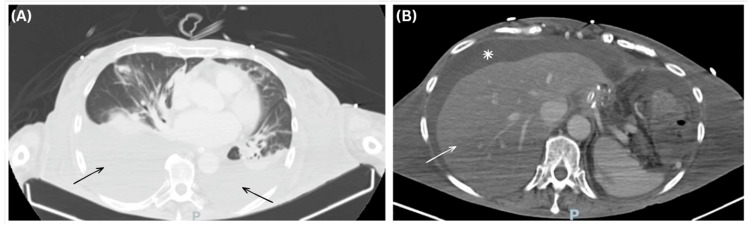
Contrast-enhanced CT demonstrating complications associated with severe hepatic decompensation. (A) Large bilateral pleural effusions (black arrows) with adjacent compressive atelectasis. (B) Hepatomegaly with diffuse hepatic steatosis (white arrow) and surrounding ascites (*).
